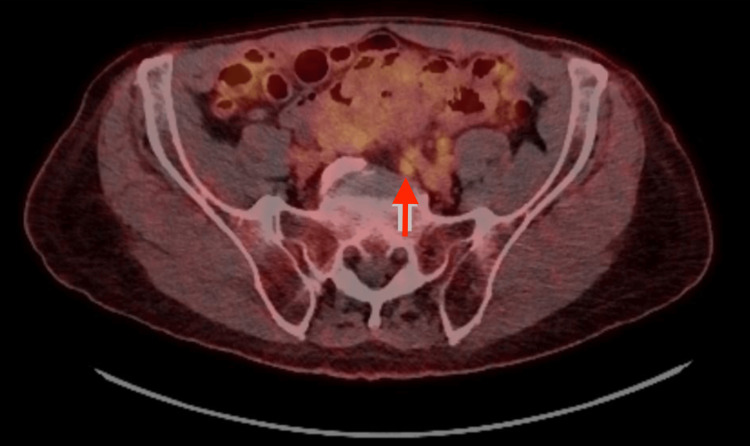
FDG-PET/CT depicting suspicious common iliac lymph node FDG: fludeoxyglucose F18; PET: positron emission tomography; CT: computed tomography
